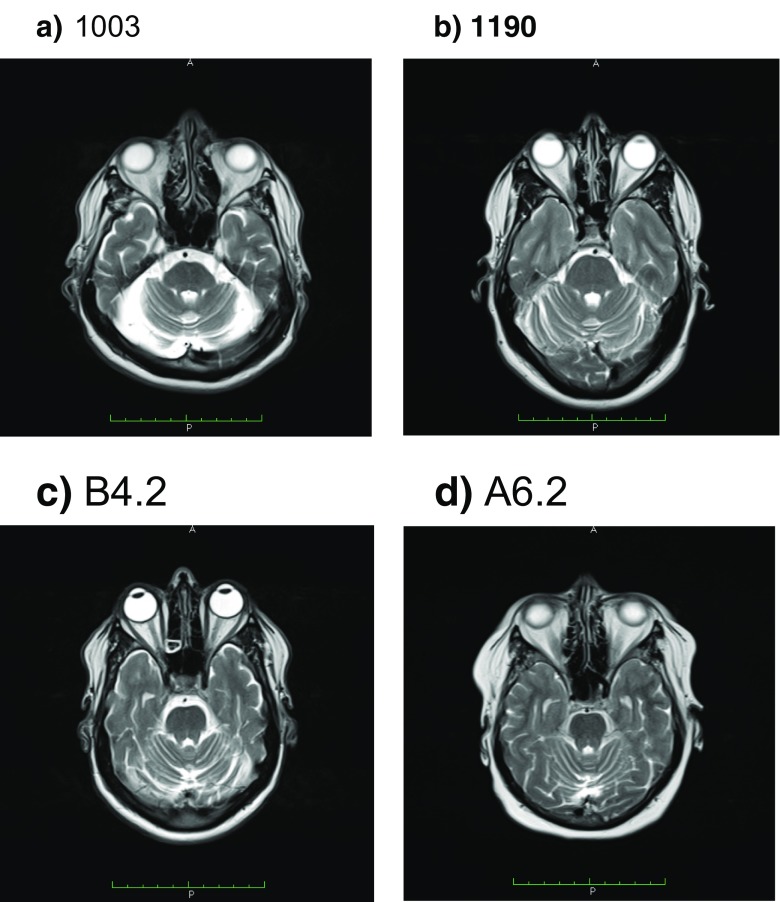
Axial T2-weighted brain MRI of the four individuals showing mild cerebellar atrophy. a CMT1003. b CMT1190. c B4.2. d A6.2
